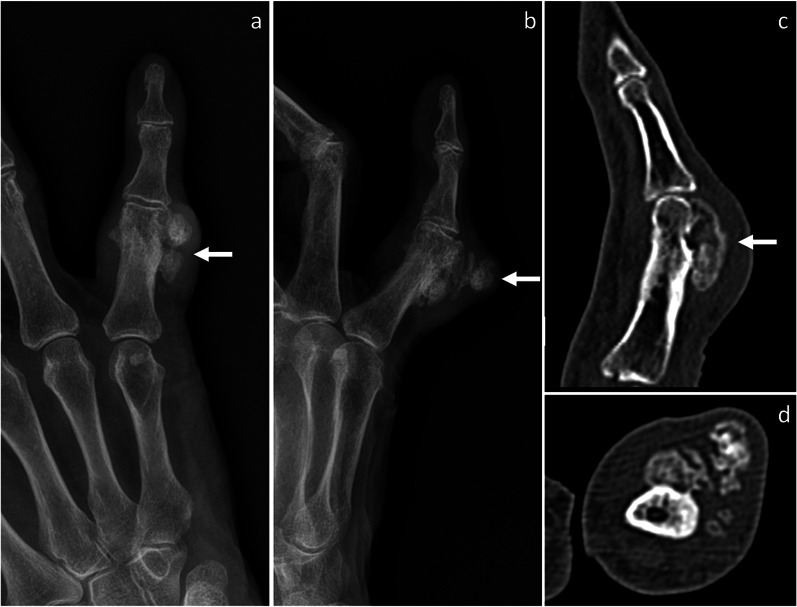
Recurred BPOP arising from the proximal phalanx of the little finger (same patient shown in Fig. 1). Eighteen months after surgery, BPOP recurrence is noted and shows more irregular mineralization compared to the original lesion, as shown on frontal (a) and oblique (b) X-rays views, as well on sagittal (c) and axial (d) CT images. Arrow points at BPOP in all images
